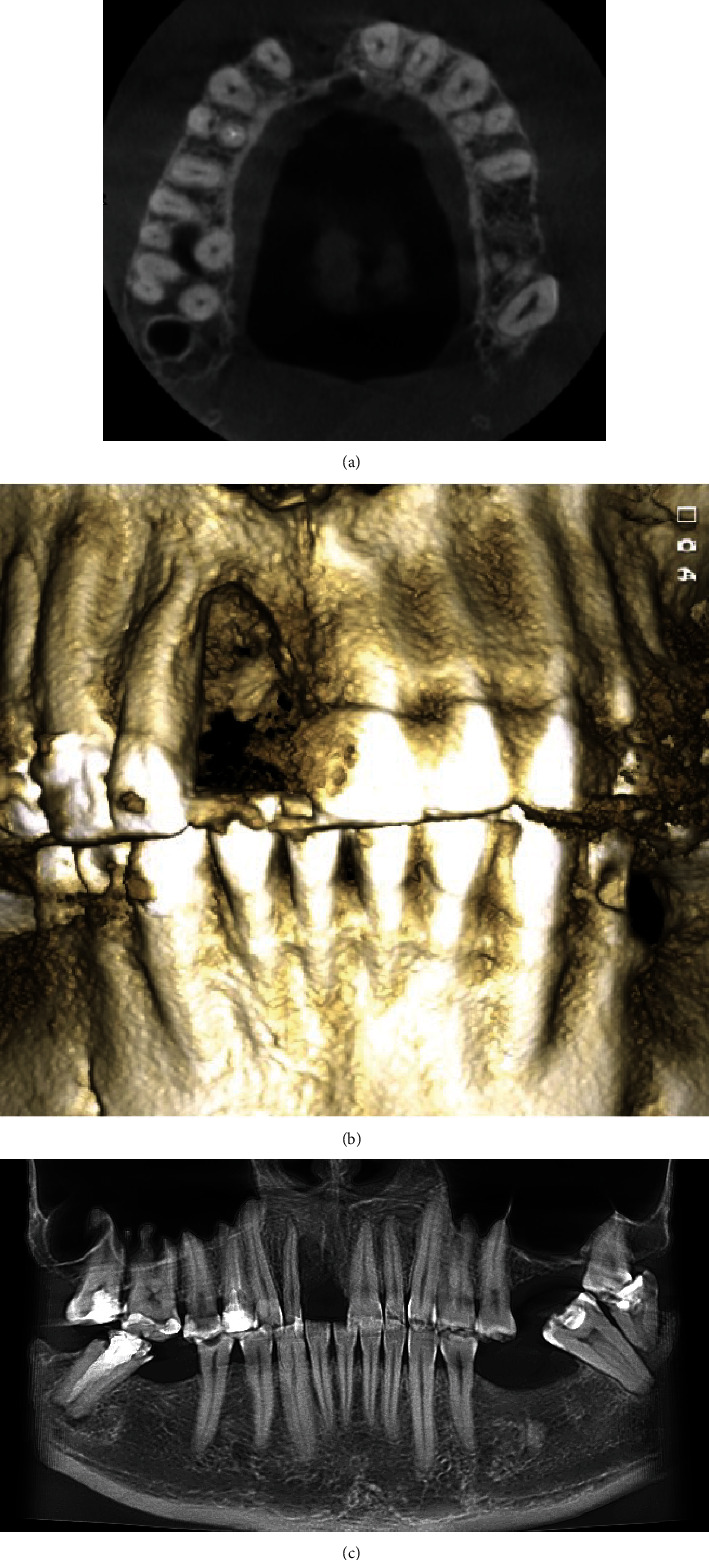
Cone-beam computed tomography scans obtained before the surgery.
